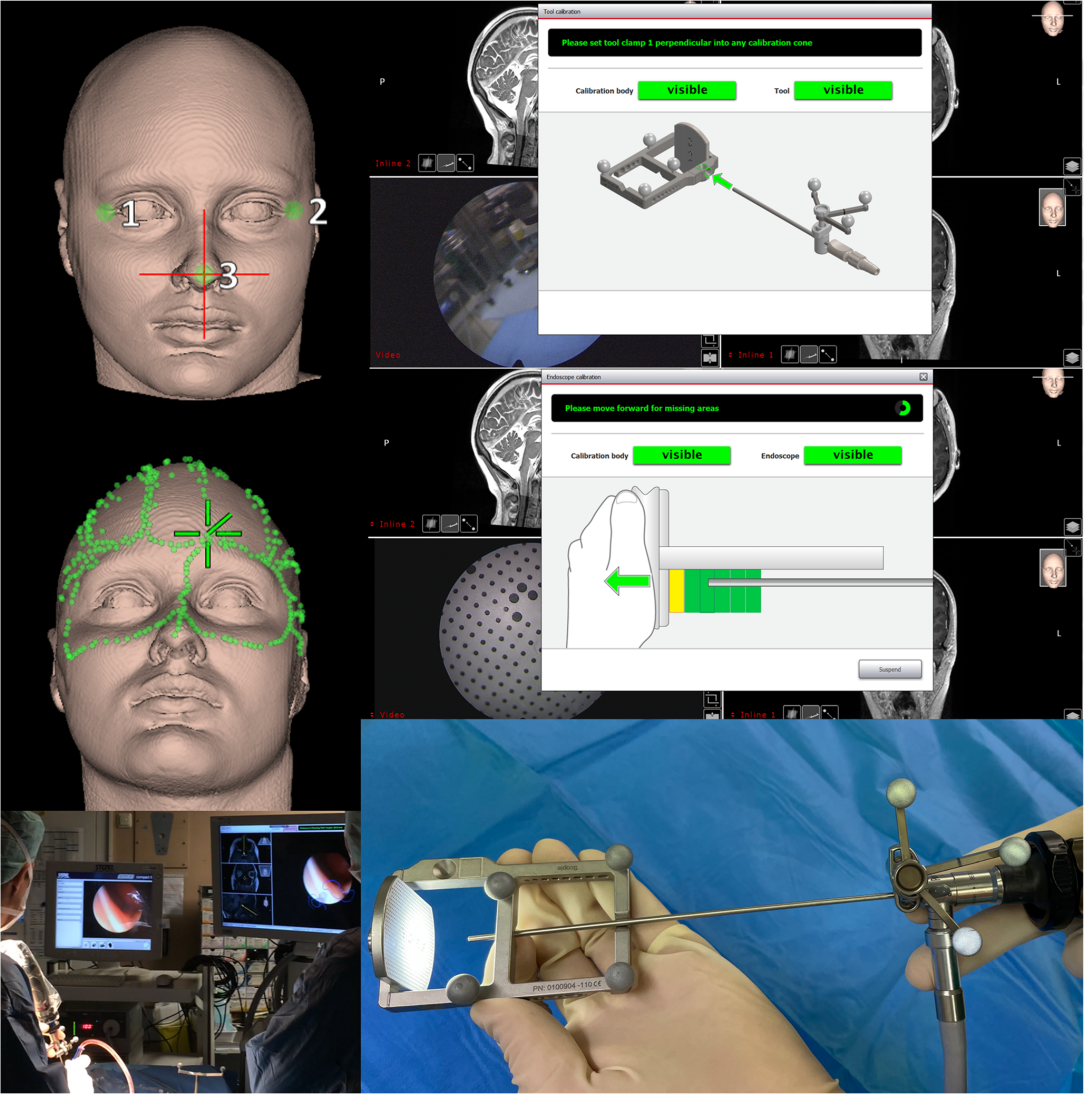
Hybrid registration procedure of the patients head before surgery capturing anatomical landmarks and multiple random points on the surface of the nose, the forehead and the anterior calvarium (left upper and middle pictures). The registration of the endoscope includes two steps using a registration matrix in which the length of the endoscope was defined and the visual field was registered at a specific magnification and camera orientation using a predefined matrix pattern (right upper, middle and lower pictures). In the intraoperative setting both, the endoscope’s and neuronavigation screen could be appreciated in parallel. The endoscopic views on the navigation screen incorporates the AR information together with the reconstructed 3D MRI sections along the endoscopes orientation (left lower picture)
